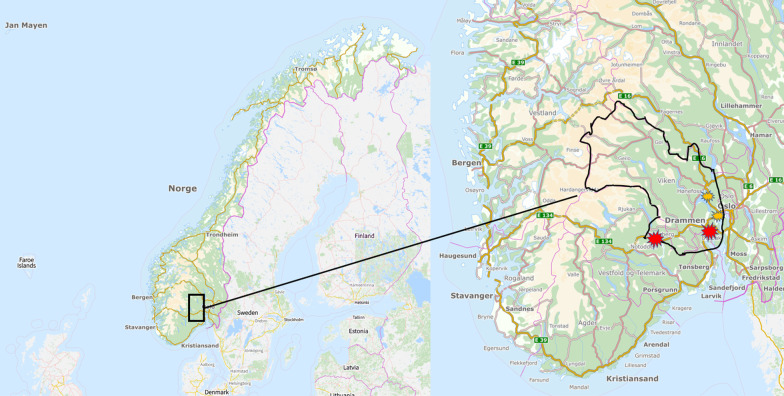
The catchment area of VVHT. The catchment area of VVHT within the black delineation. Red stars indicate locations containing both public and private providers with MRI facilities. Yellow stars indicate the location of public providers with MRI facilities
